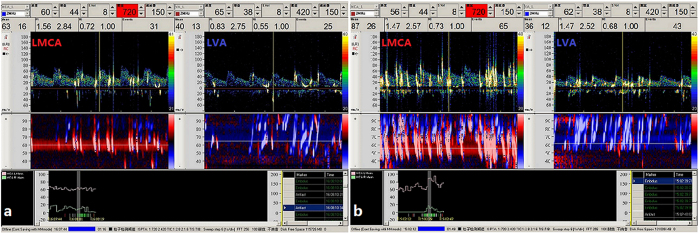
The spectra on contrast-enhanced transcranial Doppler (c-TCD) of simultaneous left middle cerebral artery (LMCA) and left vertebral artery (LVA) monitoring. (a) The spectra on c-TCD of simultaneous LMCA and LVA monitoring at rest. (b) The spectra on c-TCD of simultaneous LMCA and LVA monitoring during the Valsalva manoeuvre.
